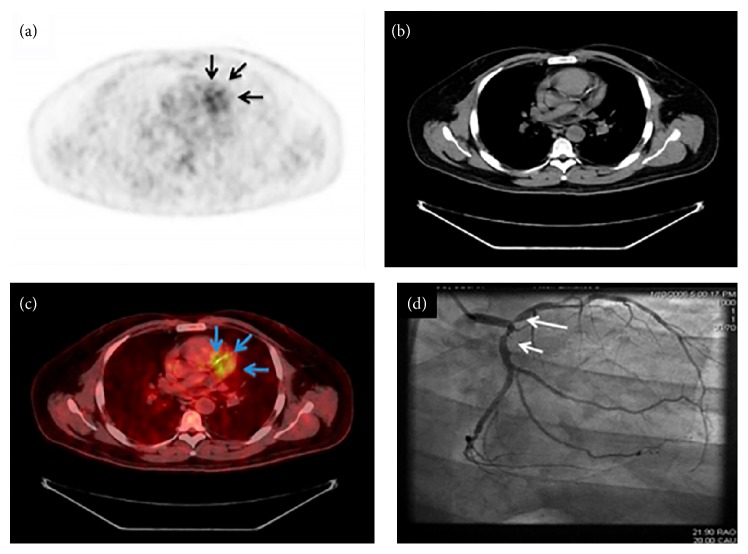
Representative images of coronary tree FDG uptake with corresponding angiographic images. Representative images of the coronary tree FDG uptake (arrows). FDG PET (a). CT (b). PET/CT (c) and coronary angiography (d) from patient with good myocardial uptake suppression with a low carbohydrate, high fat preparation. Reprinted with permission from Wykrzykowska et al. [52].
